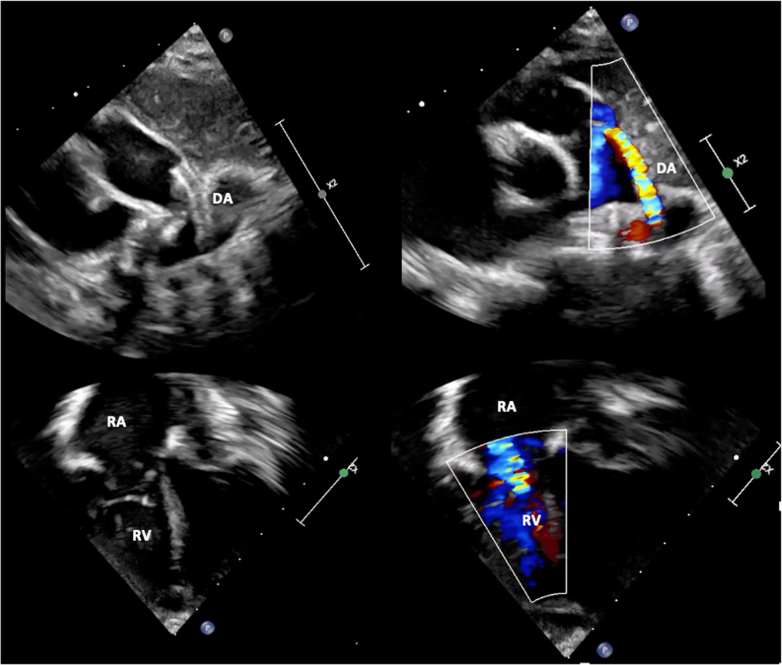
Follow-Up Transthoracic Echocardiography at Age 18 Months (Top Panels) Parasternal views showing the stent of the ductus arteriosus, with sustained retrograde flow into the pulmonary arteries. (Bottom Panels) Apical 4-chamber views demonstrating right ventricular growth and reduced tricuspid valve regurgitation, supporting successful right ventricular remodeling and progressive transition toward biventricular circulation. Abbreviations as in Figure 1.
